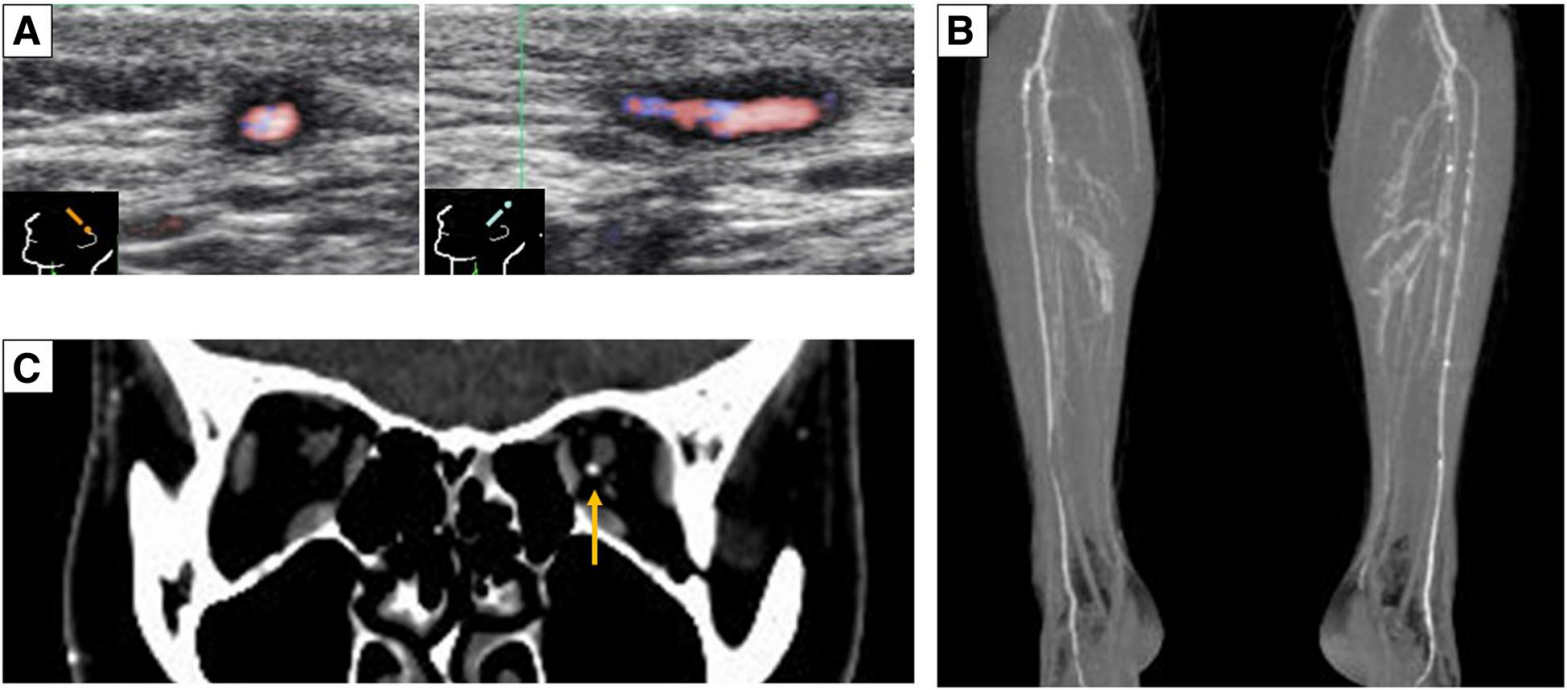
Multiple vascular stenoses and occlusion. a Vascular ultrasonography revealed concentric hypoechoic mural thickening (the halo sign) in the left temporal artery. b Reconstruction image of an enhanced computed tomography scan showed bilateral occlusion of the posterior tibial arteries and the peroneal arteries. c Enhanced computed tomography showed occlusion of the right ophthalmic artery while the left ophthalmic artery was normally detected (*yellow arrow*)
